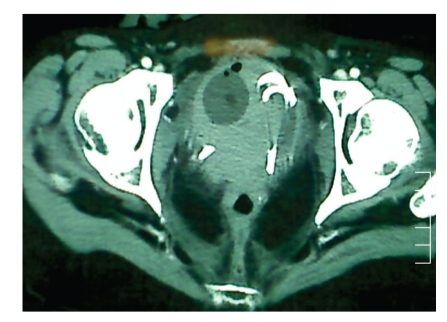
CT scan demonstrating bladder completely filled with blood clots, catheter balloon, and ureteral stents.
